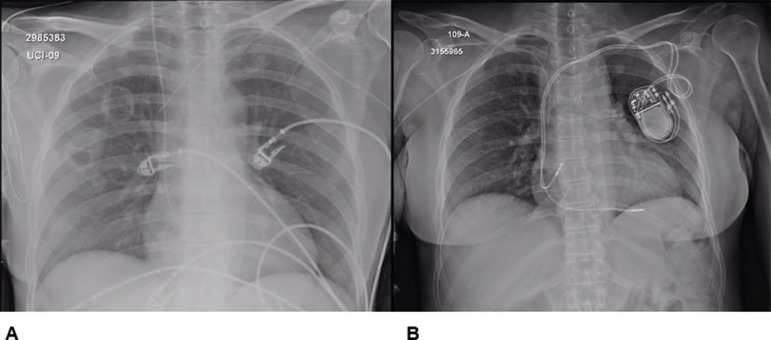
(A) Chest radiography in AP view showing bilateral nodules of the entry
